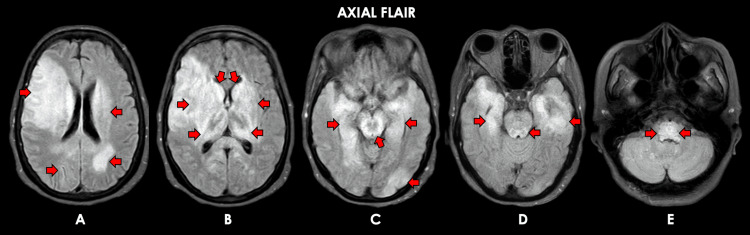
Representative cranial MRI sequences for patient #2. Cranial MRI obtained approximately 2 months after the onset of illness. Representative axial brain MRI (FLAIR sequence) images show confluent, non–contrast-enhancing, ill-defined T2-FLAIR–hyperintense lesions in the bilateral frontoparietal cortical, subcortical, and periventricular regions (A); bilateral insular cortex, caudate nuclei, thalami, and lentiform nuclei (B, C); bilateral medial temporal lobes, midbrain, and pons (D); and bilateral medulla (E). FLAIR: Fluid-attenuated inversion recovery; DWI: Diffusion-weighted imaging.
